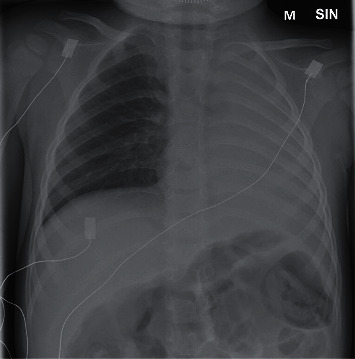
Follow-up chest X-ray 12 h after arrival. The left lung is completely atelectatic, and the mediastinum has shifted to the left.
